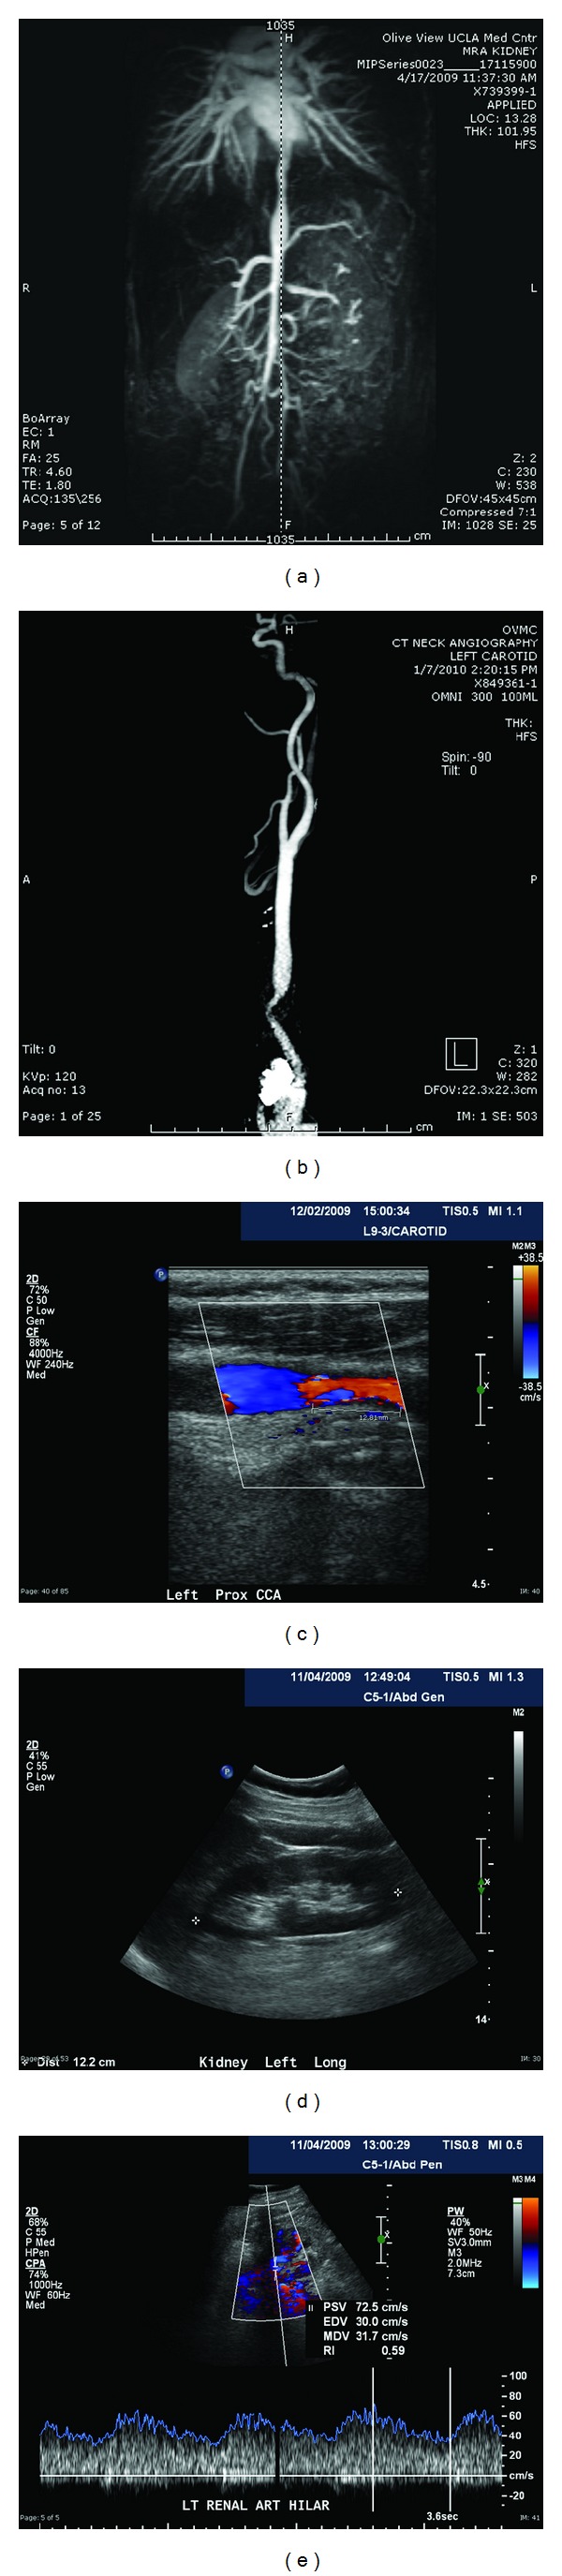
images of patient Y.P. and Takayasu's arteritis (a) Patient Y.P. MRA of abdomen and pelvis from 04/01/2009. 3D angiogram showing thoracic aortic stenosis at the level of the descending aorta and abdominal aortic stenosis. Stenosis of the left renal artery is also identified. (b) Patient Y.P. Carotid CT-Angiogram of the neck from 01/07/2010. 3D angiogram showing striking left common carotid artery stenosis. (c) Patient Y.P. Carotid Ultrasound with Doppler flow imaging from 12/02/2009. Figure shows a severe left common carotid artery stenosis of 70%. (d) Patient Y.P. renal ultrasound from 11/04/2009. Figure shows a normal sized left kidney measuring 12.2 cm. (e) Patient Y.P. renal ultrasound with Doppler flow imaging from 11/04/2009. Figure shows left renal artery stenosis by Doppler velocity measurement showing an abnormal resistive index of 0.59 and velocity of 182 cm/s fulfilling one of two criteria for left renal artery stenosis.
